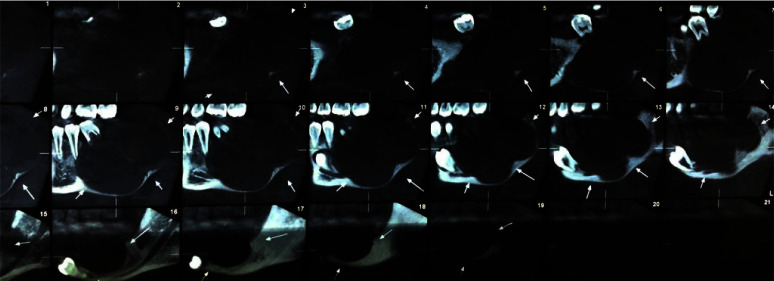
Sagittal view of the lytic lesion in CBCT
